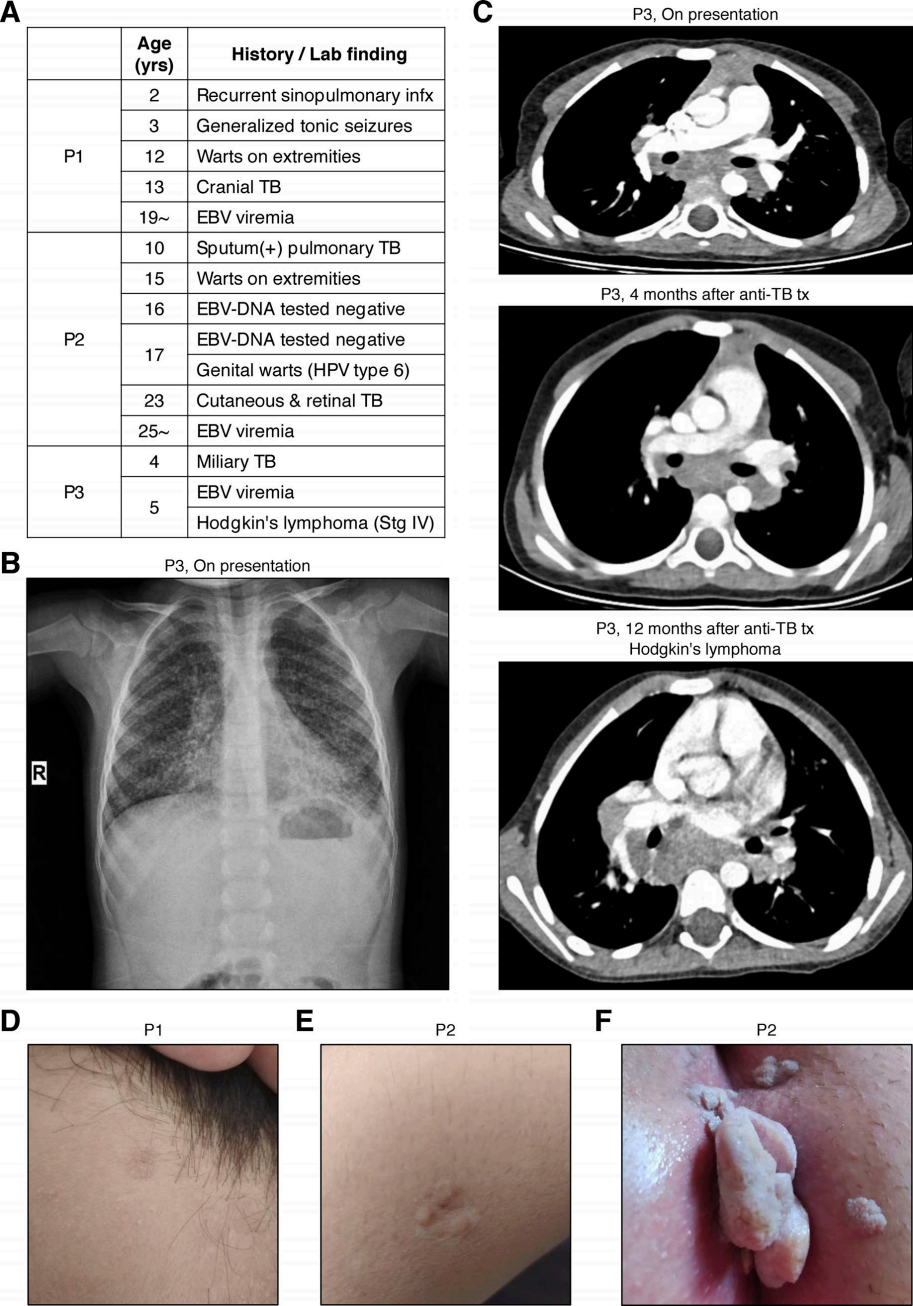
Clinical findings. (A) A table summarizing medical history and key laboratory findings related to infectious diseases in the three patients studied. (B) Chest x ray of P3 on presentation. (C) Thoracic CT scans of P3. (D) A common wart on the forehead of P1. (E) A common wart on the extremity of P2. (F) A genital wart in P2. Infx, infections; Stg, stage.
